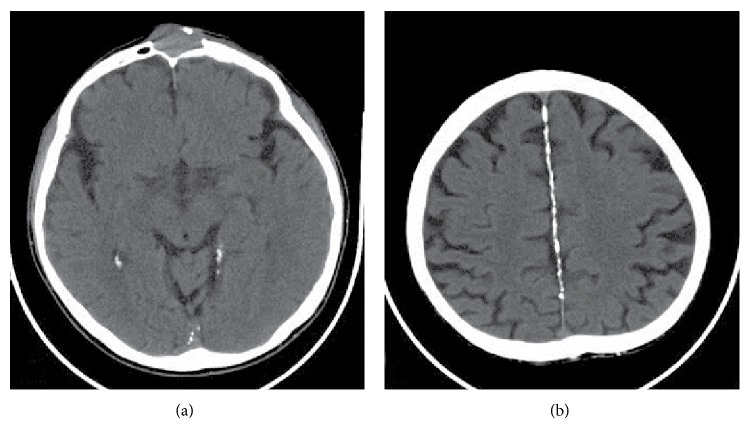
CT imaging of brain: (a) calcifications at tentorium and mass at frontal sinus with bone destruction and expansion. (b) Diffuse calcification at falx cerebri.
